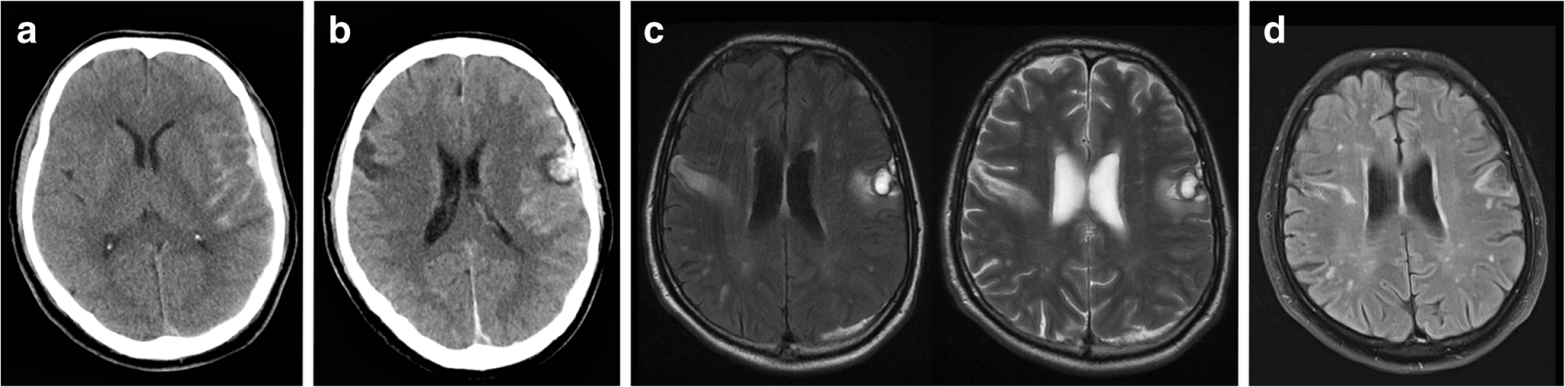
Neuroradiological imaging. CT scan on the day of the injury (a) and 2 days later (b). MRI scan performed at 2 weeks demonstrated bilateral opercular lesions (c). Progress MRI at 13 months demonstrated persistent FLAIR signal change in these regions (d)
